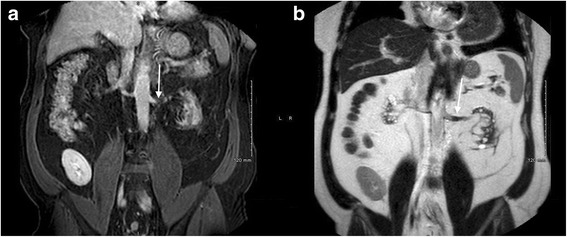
a Coronal MR angiography demonstrating an abrupt cessation of flow in the left renal artery (*white arrow*). b Coronal T1-weighted MRI from the same patient showing the device (*white arrow*) in situ within the left renal artery. Note the lack of susceptibility artefact
